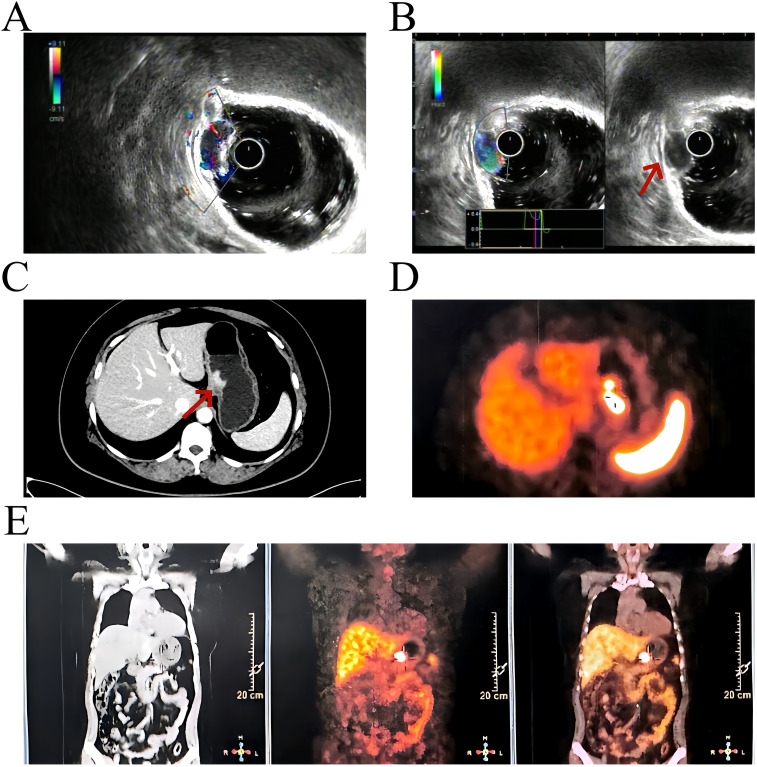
Diagnostic imaging. (A, B) Endoscopic ultrasound revealed two distinct lesions: a hypoechoic mass measuring 23.3 × 16.9 mm, located at the esophagogastric junction, involving the mucosal and submucosal layers (red arrow) with relatively abundant blood flow signals and firm consistency; and a separate homogeneous polypoid lesion in the gastric body confined to the mucosa. (C) Abdominal CT shows an intraluminal protruding mass at the esophagogastric junction (red arrow), which demonstrates marked heterogeneous enhancement in the arterial phase and homogeneous enhancement in the portal venous phase on contrast-enhanced scan. (D, E) 68Ga−DOTATATE PET/CT reveals wall thickening and an intraluminal protruding mass at the esophagogastric junction, with corresponding abnormal radiotracer avidity on PET. No significant abnormal metabolic activity is observed elsewhere in the body.
